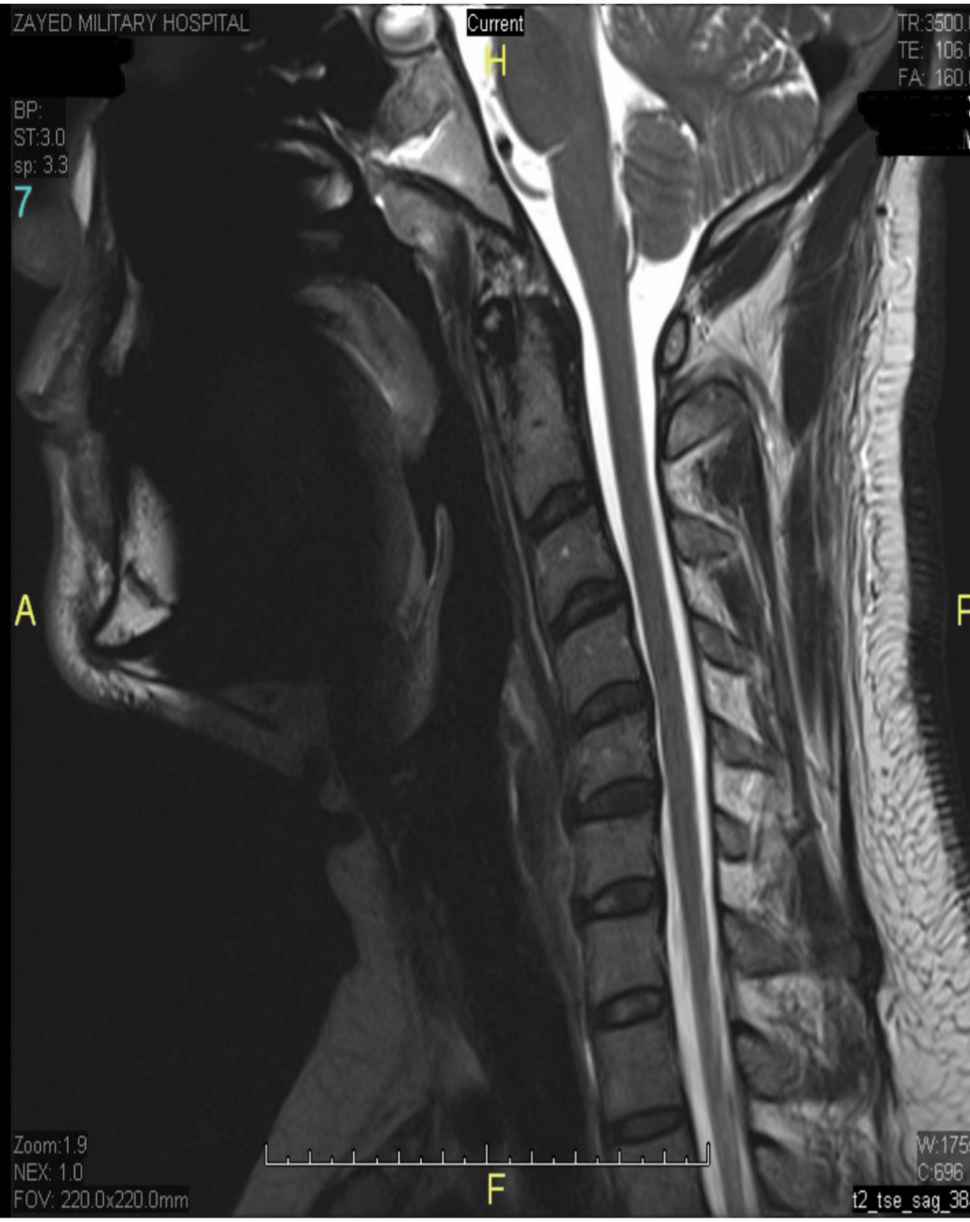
Repeat Sagittal T2-Weighted MRI Demonstrating Resolution of Dorsal Column Hyperintensity Repeat sagittal T2-weighted MRI of the cervical spine shows resolution of the previously noted bilateral dorsal column hyperintensity. No residual signal abnormality is seen, indicating radiological improvement following vitamin B12 replacement.
